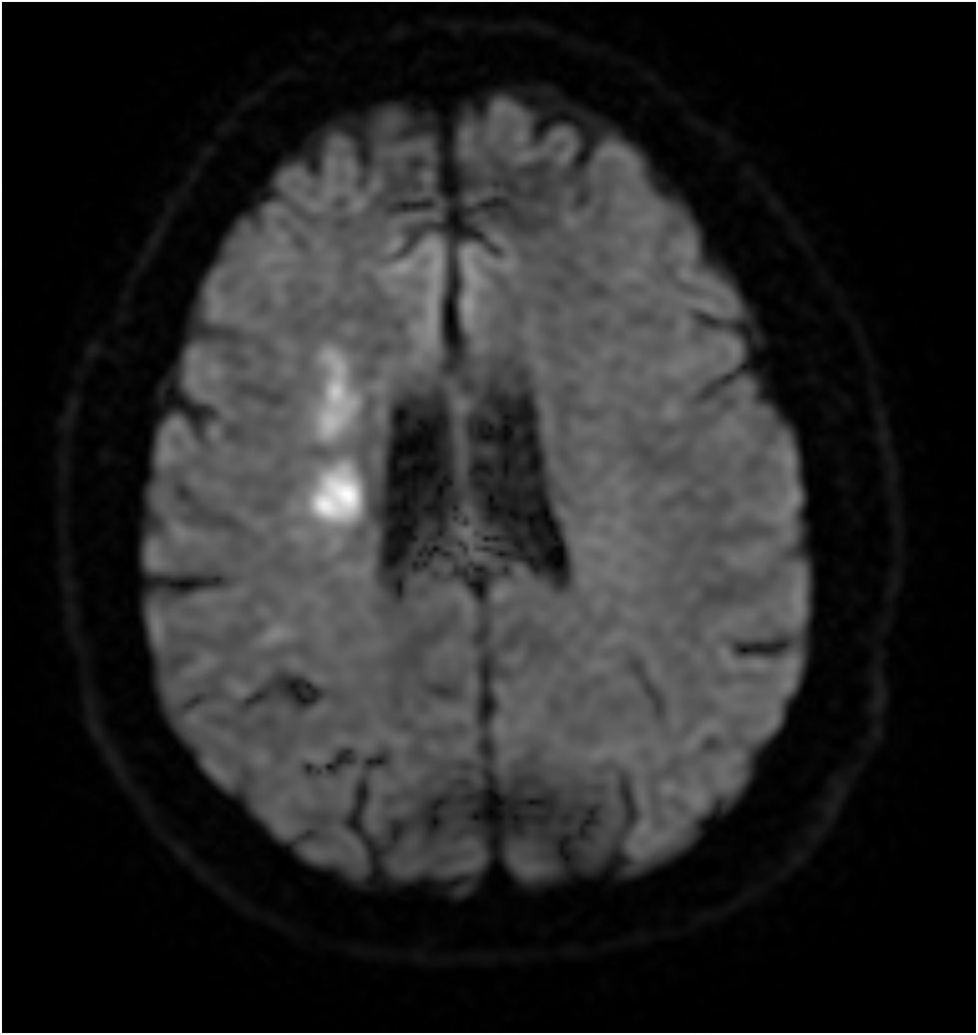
MRI DWI sequence of Borderzone Infarct.
